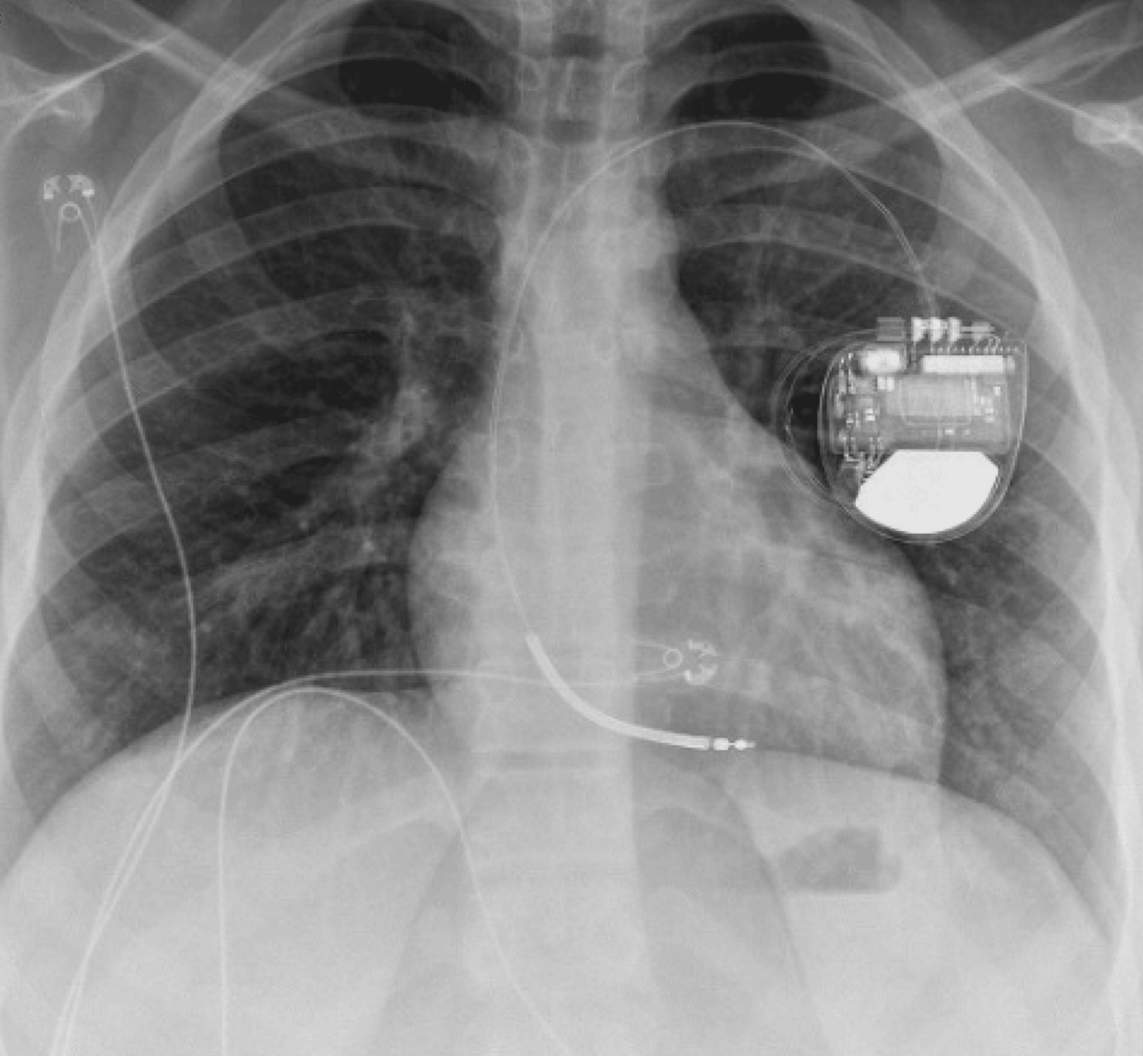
A chest X-ray confirming successful implantation of the single-chamber transvenous implantable cardioverter defibrillator.
